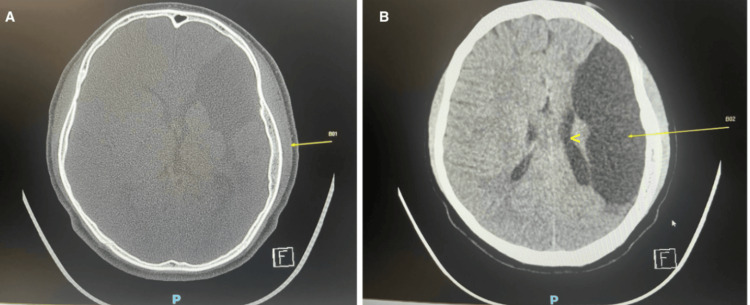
Non-contrast CT of the brain demonstrating features of Dyke-Davidoff-Masson syndrome. (A) Axial CT image demonstrating left cerebral hemiatrophy with ipsilateral calvarial thickening. (B) Axial CT image at the level of the lateral ventricles demonstrating extensive left frontoparietal encephalomalacia (arrow) involving left middle cerebral artery territory with associated ex vacuo dilatation of the left lateral ventricle (arrowhead).
